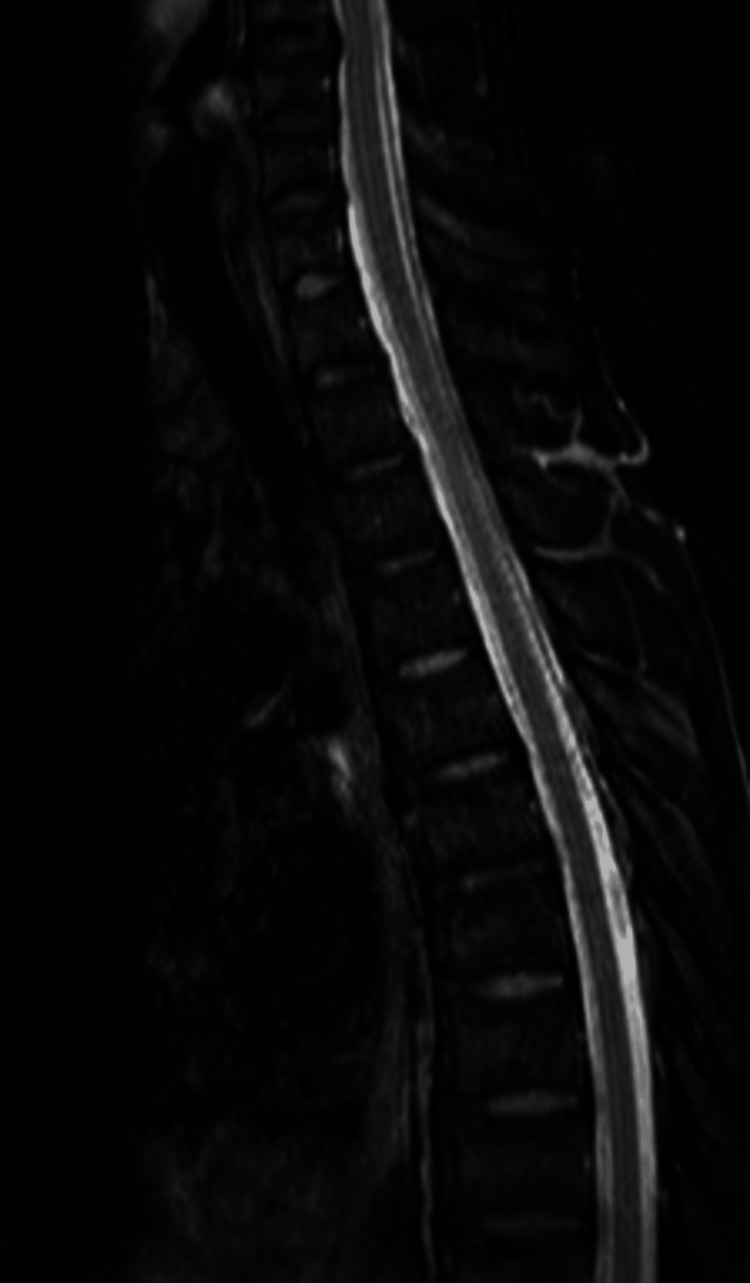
Magnetic resonance imaging after the third epidural blood patch. Sagittal T2-weighted image shows no extradural cerebrospinal fluid collection.
